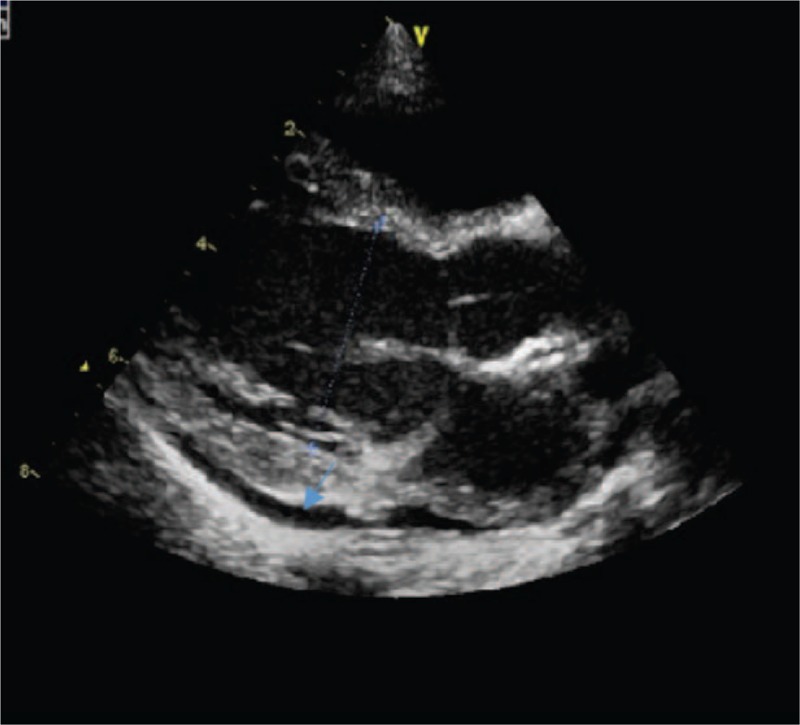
Echocardiography showed a small amount of pericardial effusion (PE).
